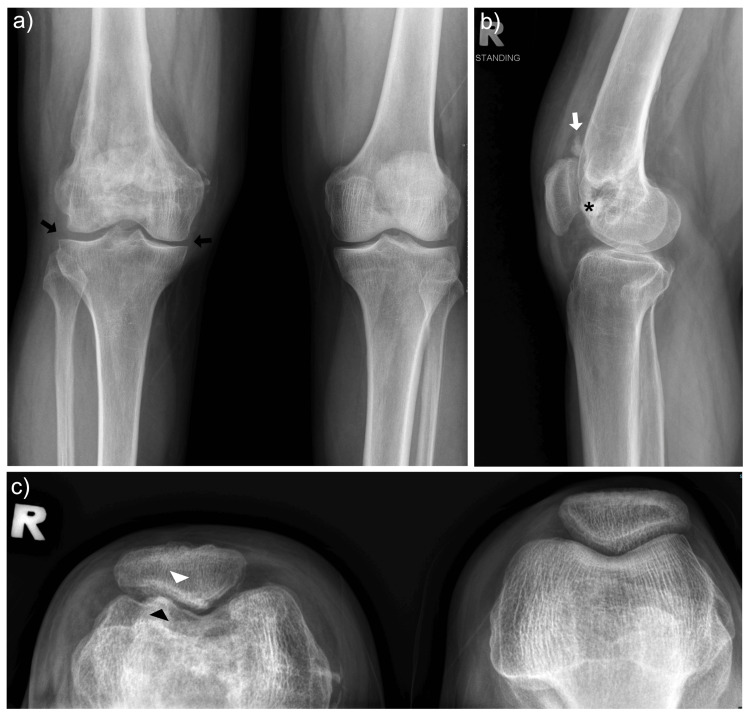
Pre-operative radiographs of the right knee (a) Standing anteroposterior (AP) view showing preserved medial and lateral tibiofemoral joint spaces (black arrow)
